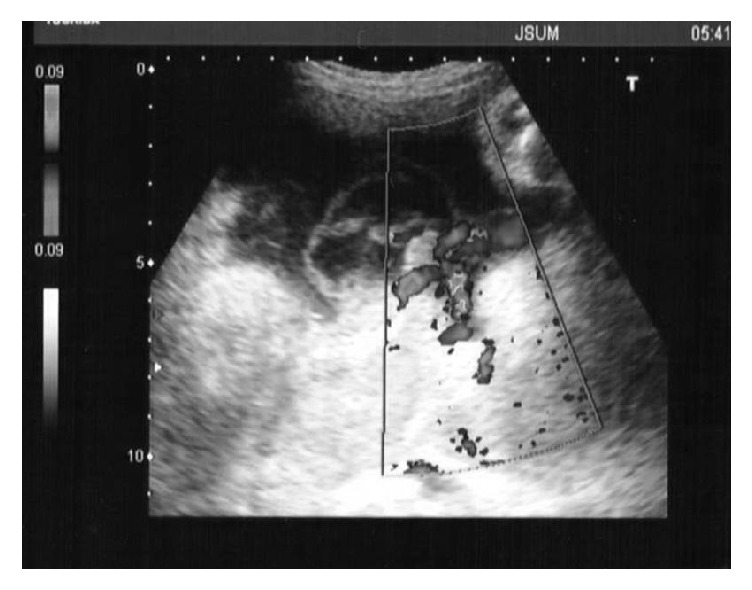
Ultrasonographic image showing subamniotic hematoma at 27 weeks in Case 4.
